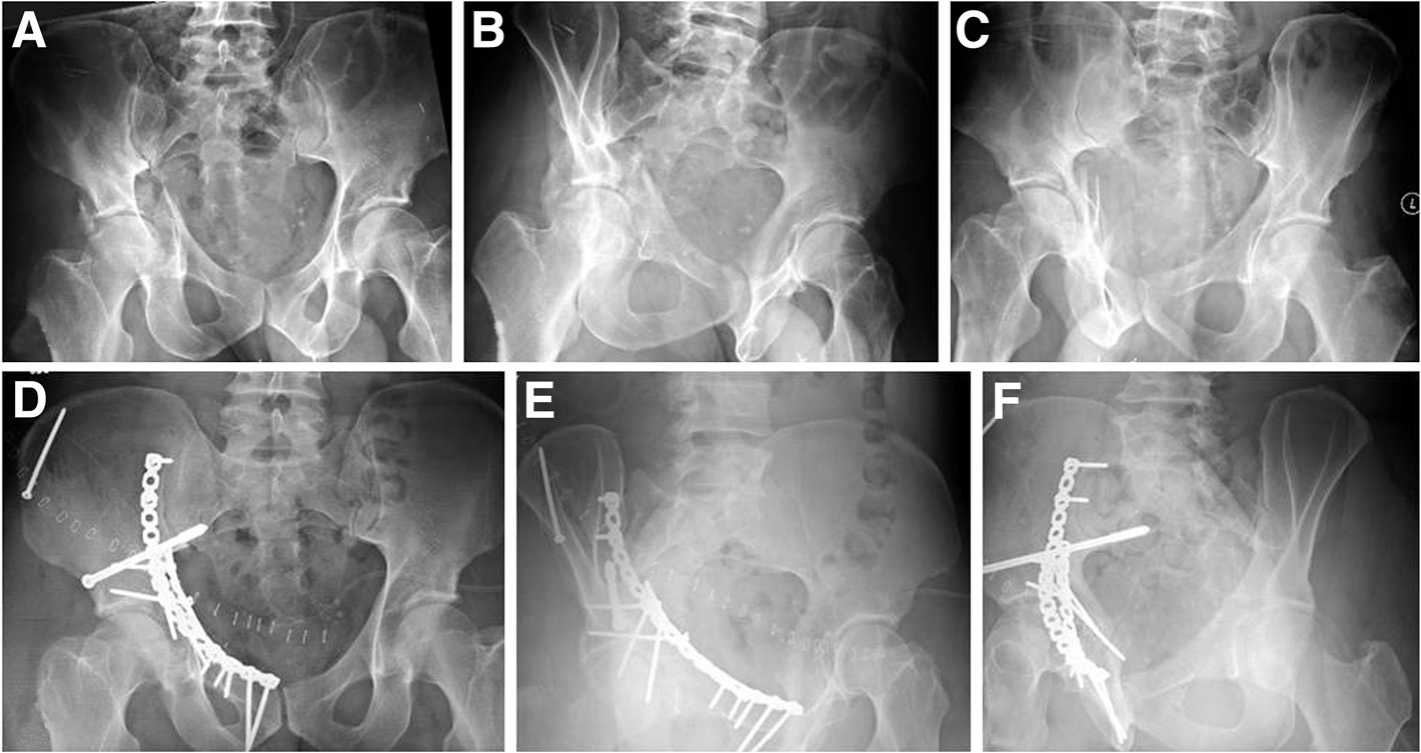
Example of an anterior column and posterior hemitransverse fracture treated through a modified ilioinguinal approach, using 3.5 mm reconstruction plates and a 7.3 mm cannulated screw. (A-C) Preoperative X-rays (a.p., obturator oblique, iliac oblique). (D-F) Postoperative X-rays (a.p., obturator oblique, iliac oblique).
